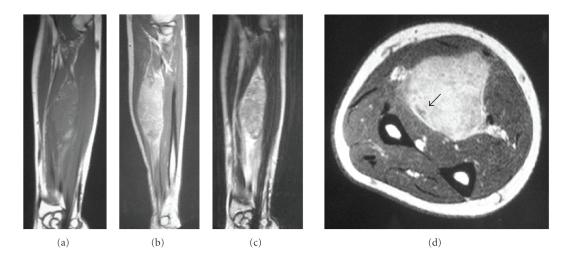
(a) Coronal T1-weighted, (b) T2-weighted, and (c)
